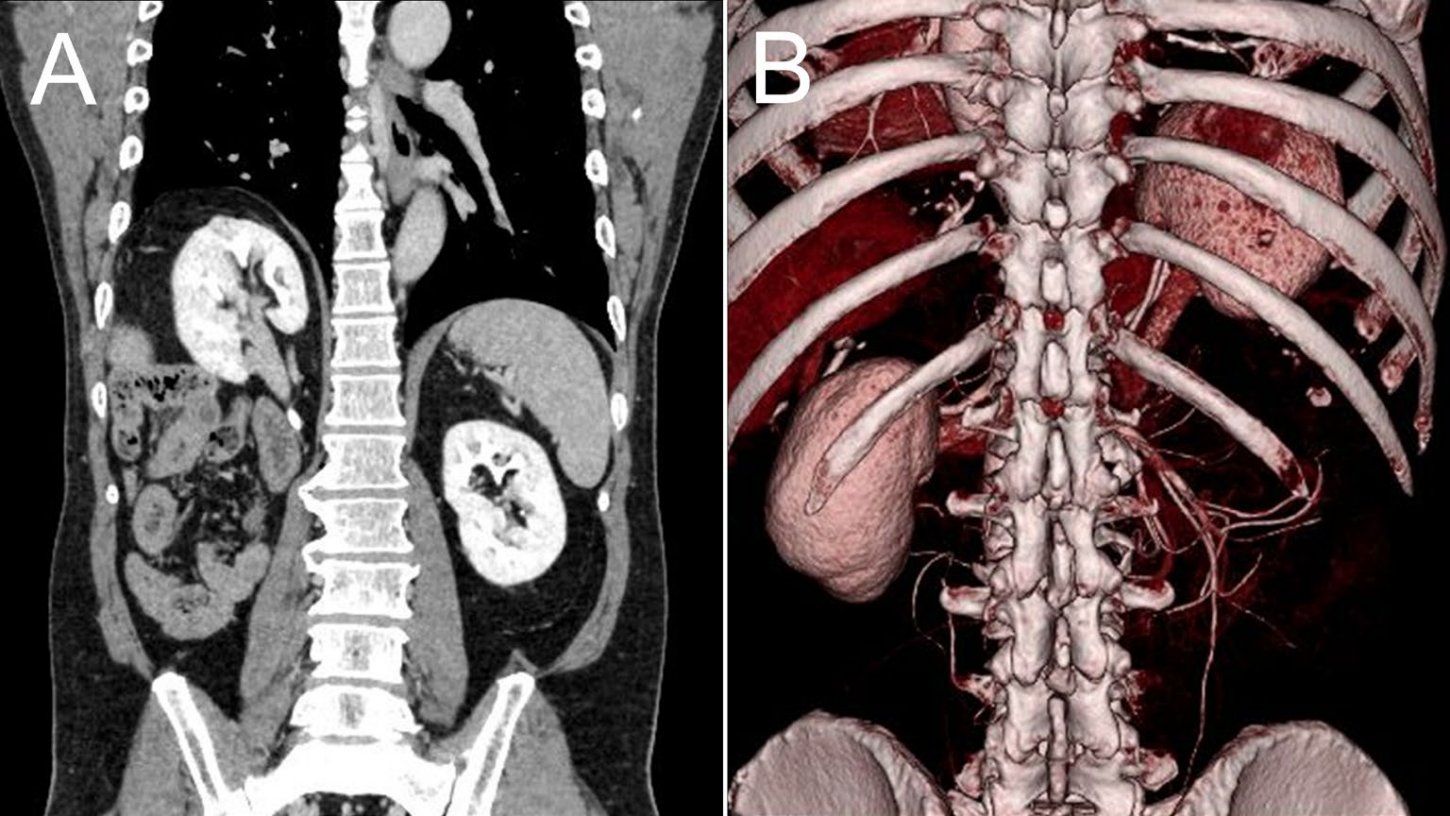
Imaging demonstrating cranial displacement of the right kidney. (A) Coronal computed tomography view showing the right kidney displaced into the thoracic cavity, reaching the level of the heart. (B) Three‐dimensional reconstructed computed tomography image clearly demonstrating the intrathoracic location of the right kidney.
